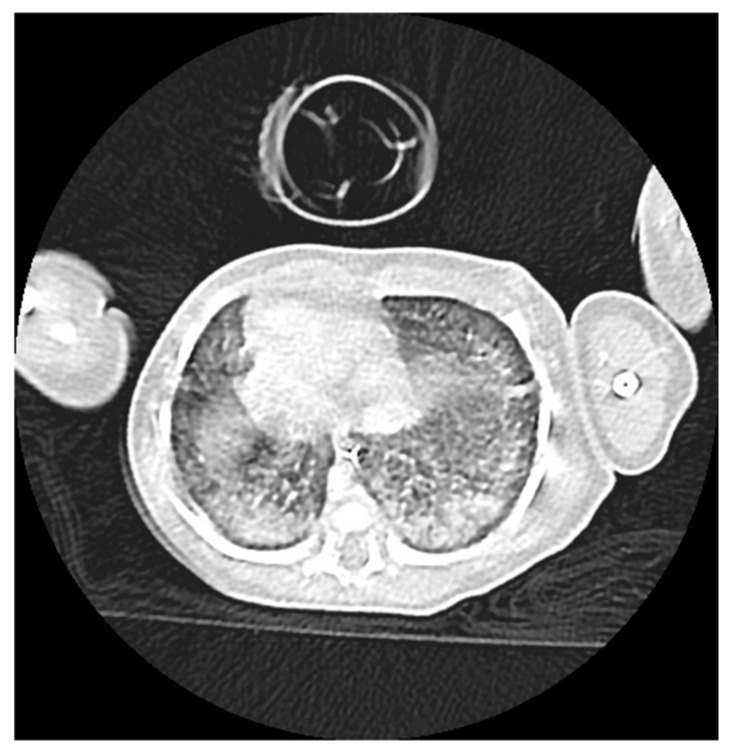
Chest computed tomography (CT) demonstrating bilateral diffuse ground-glass opacities with relative subpleural sparing.
